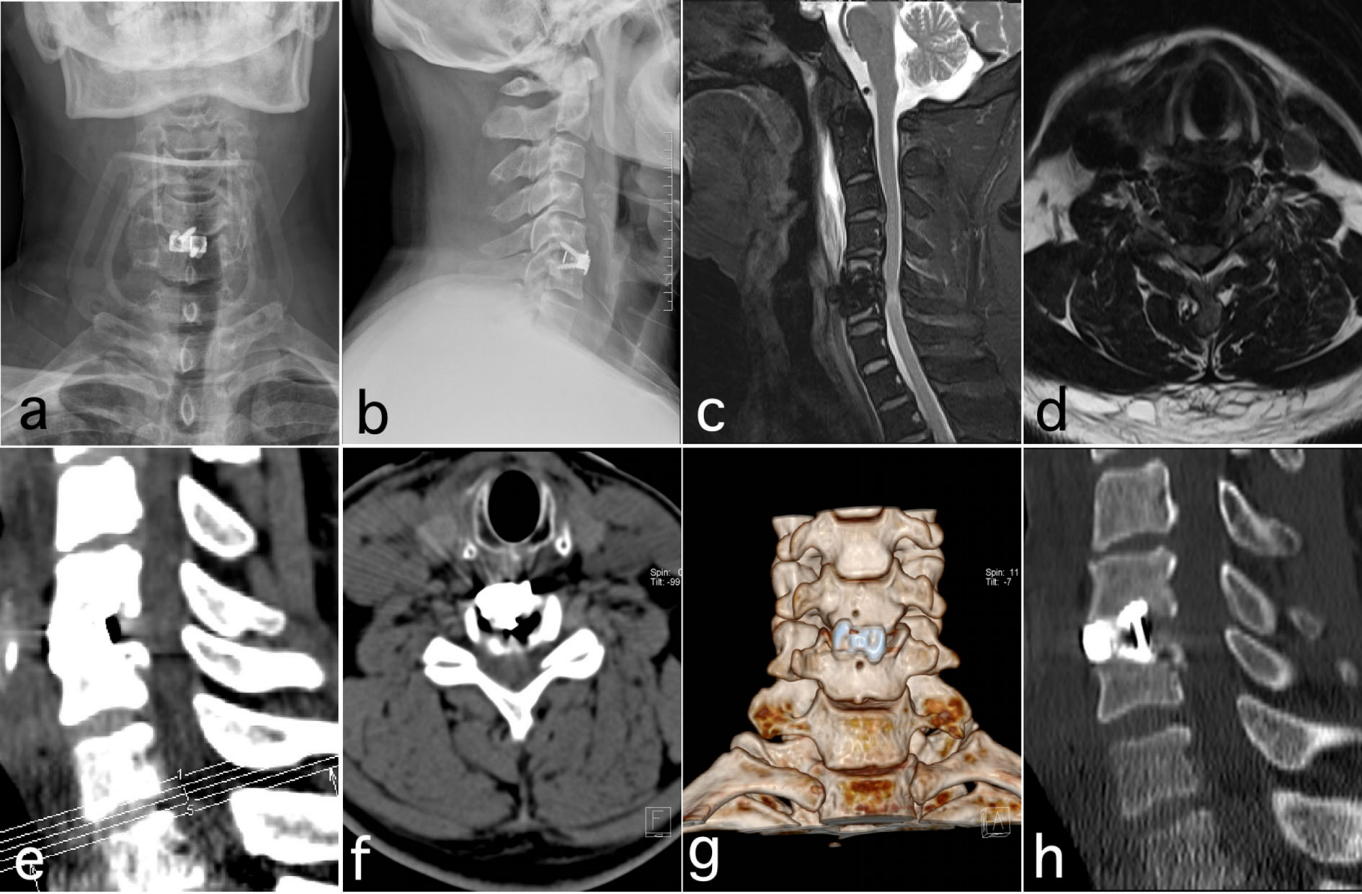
Postoperative review images of Endo-ACDF for CSM at 1 week. (a, b) X-ray shows the cage in a good position; (c-f) Postoperative MRI and CT showed effective decompression of the spinal cord and spinal canal; (g, h) Postoperative CT 3D reconstruction showed the cage in a proper position and at an appropriate depth.
